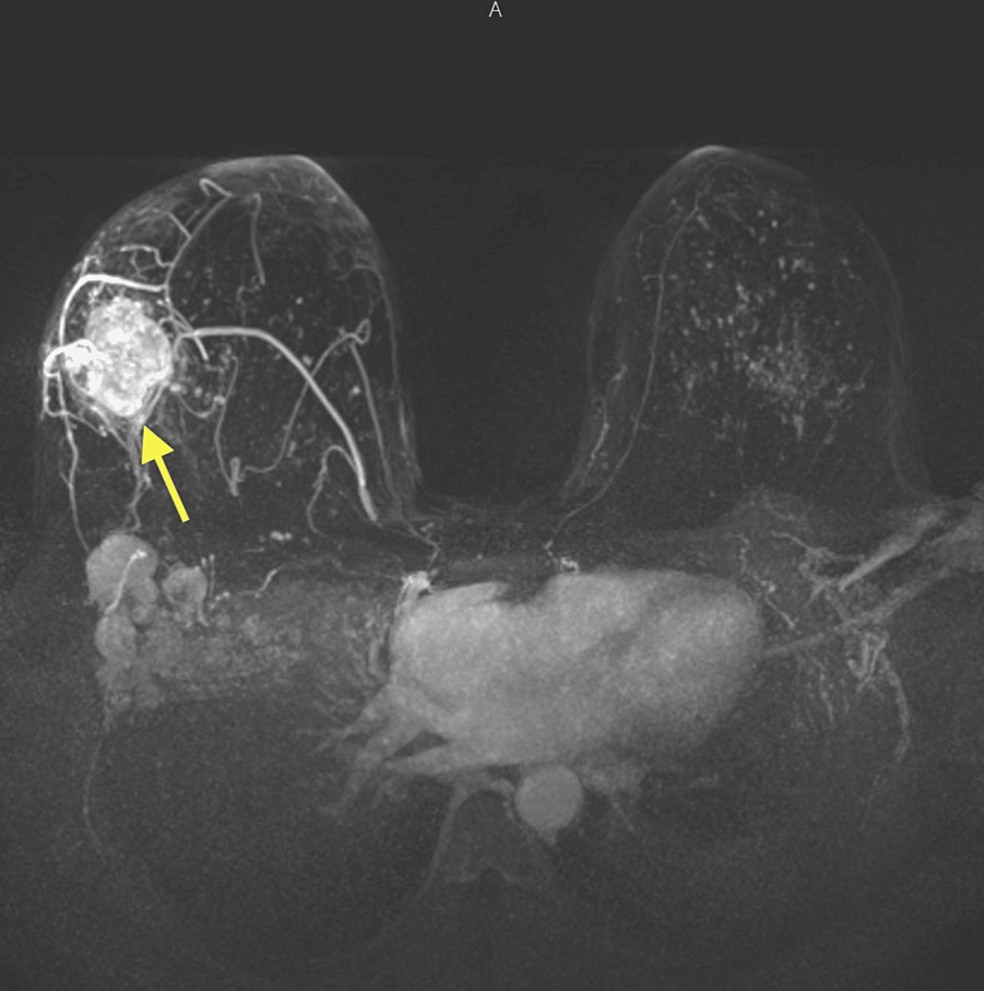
MRI right breast showing the breast mass.
